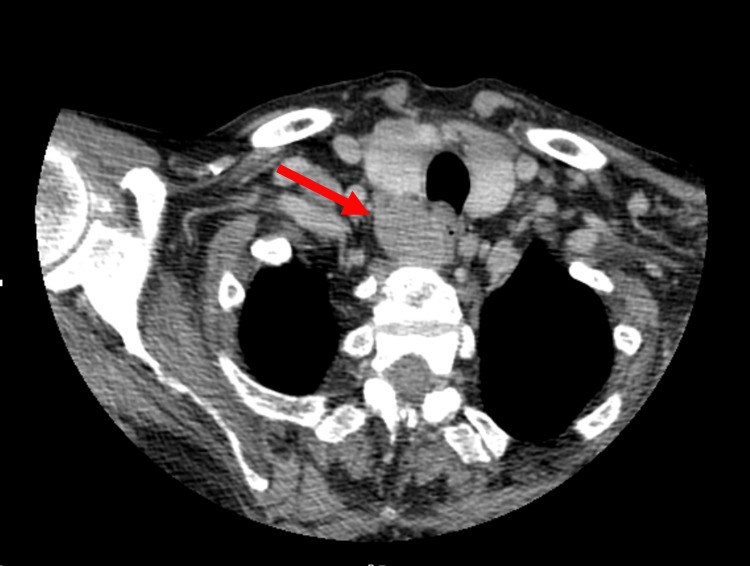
CT image showing the cervical lesion posterior (arrow) to the right thyroid lobe, measuring 31x24 mm and without apparent cleavage plane with the thyroid or esophagus.
